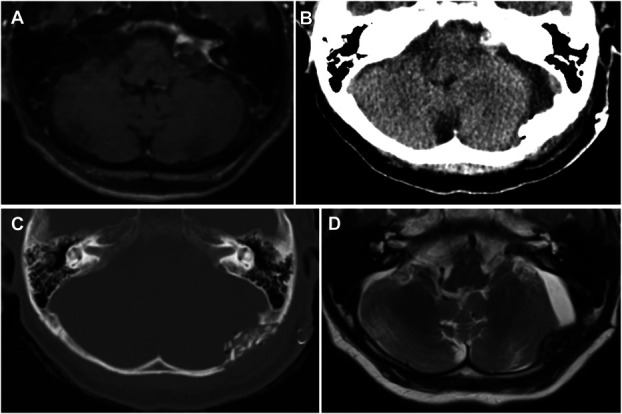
Postoperative imaging of Patient No. 19 with a left vestibular schwannoma, demonstrating no CSF leakage. A, Preoperative gadolinium-enhanced axial MRI showing a contrast-enhancing tumor in the left cerebellopontine angle. B, Noncontrast computed tomography on postoperative day 1 without evidence of CSF leakage. C, Bone window computed tomography on postoperative day 1 showing no abnormal findings. D, T2-weighted MRI on postoperative day 76 confirming absence of CSF leakage. CSF, cerebrospinal fluid.
